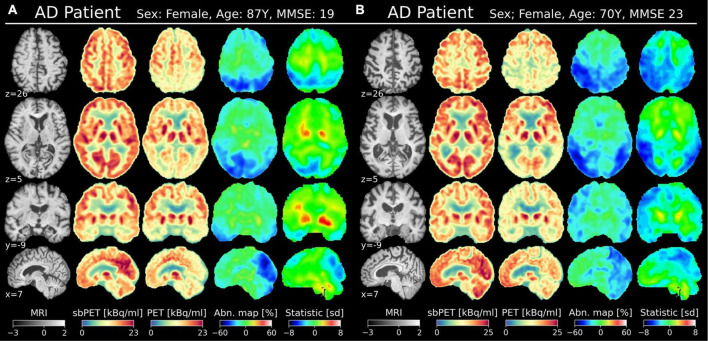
Two AD subjects in MNI space (affine transformation); one with minimal atrophy (A) and one with substantial atrophy (B). The MNI coordinate is denoted for each view. Columns from the left: T1w MRI (normalized intensity), sbPET (post-normalized), true PET, abnormality map, vendor-provided statistical map.
